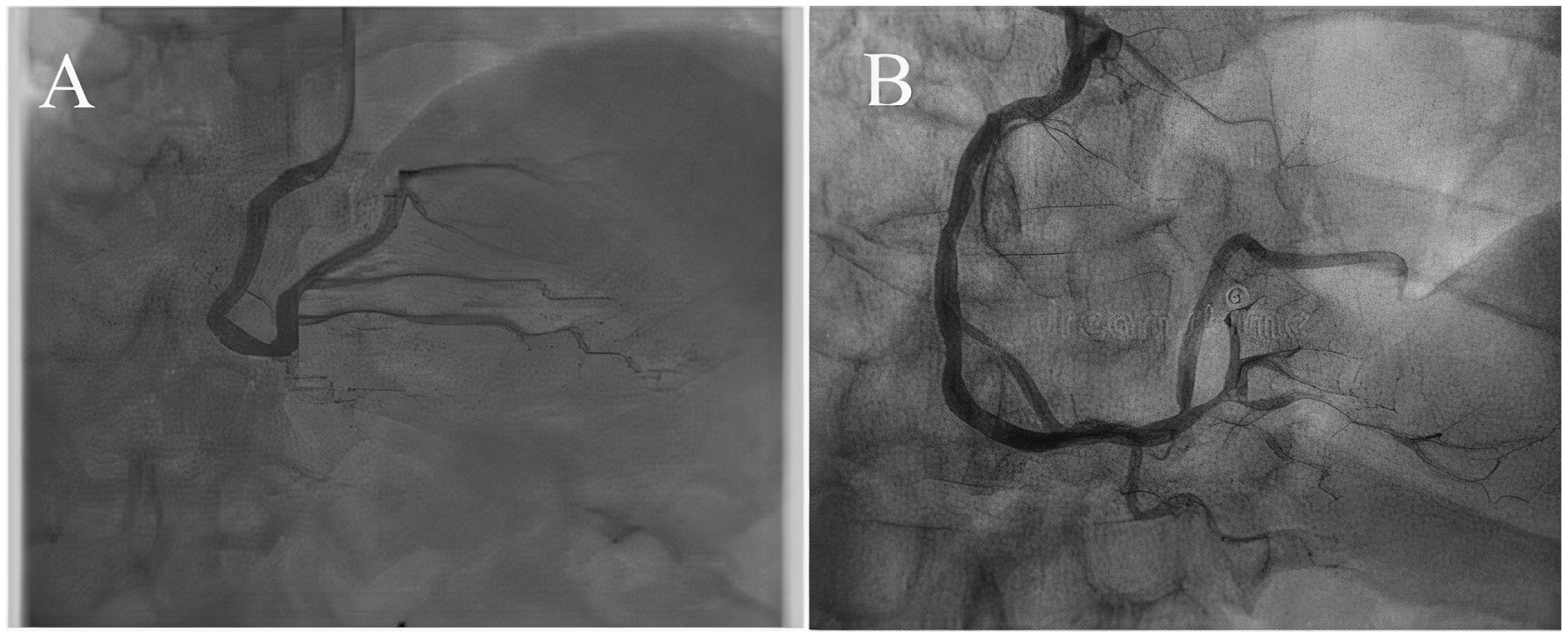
(A) and (B) X-ray angiography with noise and artefacts.
